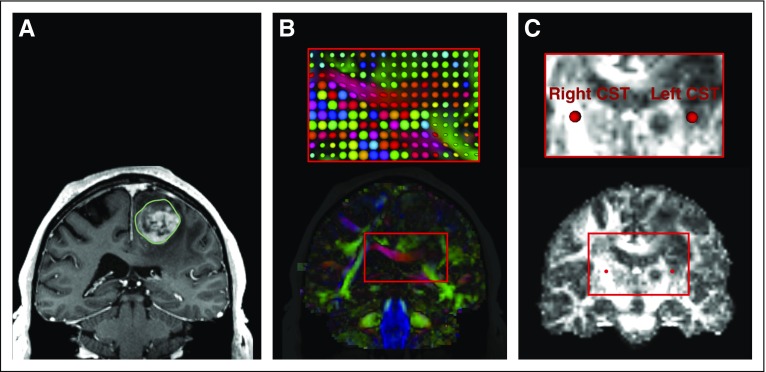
Diffusion tensor imaging (DTI) visualization and quantification in patient data set 1. The tumor-related edema and mass effect have distorted the local anatomy, including the fractional anisotropy (FA) map. (A) T1-weighted magnetic resonance imaging (MRI) with a contrast-enhancing lesion within the left frontal gyrus with the tumor volumetrically segmented in green. (B) Visualization of DTI images using a directionally encoded color map. A zoomed-in region (rectangle) is provided with a visualization using glyphs. Note that the red glyphs in the corpus callosum in the tumor-bearing hemisphere are rounder than those in the contralateral hemisphere, which indicates increased isotropic diffusion as a result of edema. (C) FA map with two fiducials in the corticospinal tract (CST; zoomed-in region outlined by the rectangle), where the FA value from the tumor-bearing hemisphere is 0.34 and that from the healthy hemisphere is 0.84.
